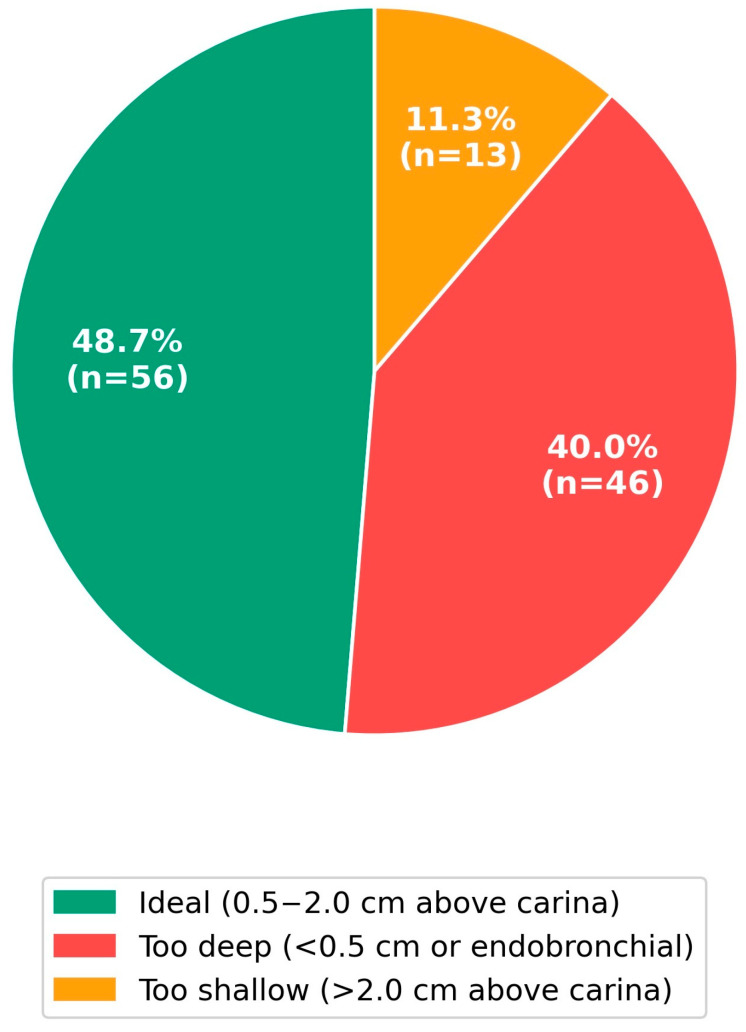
Radiographic ETT tip position at the time of the first post-intubation chest X-ray (n = 115). The ETT tip position was classified based on the final consensus tip-to-carina distance, independent of any formula prediction.
